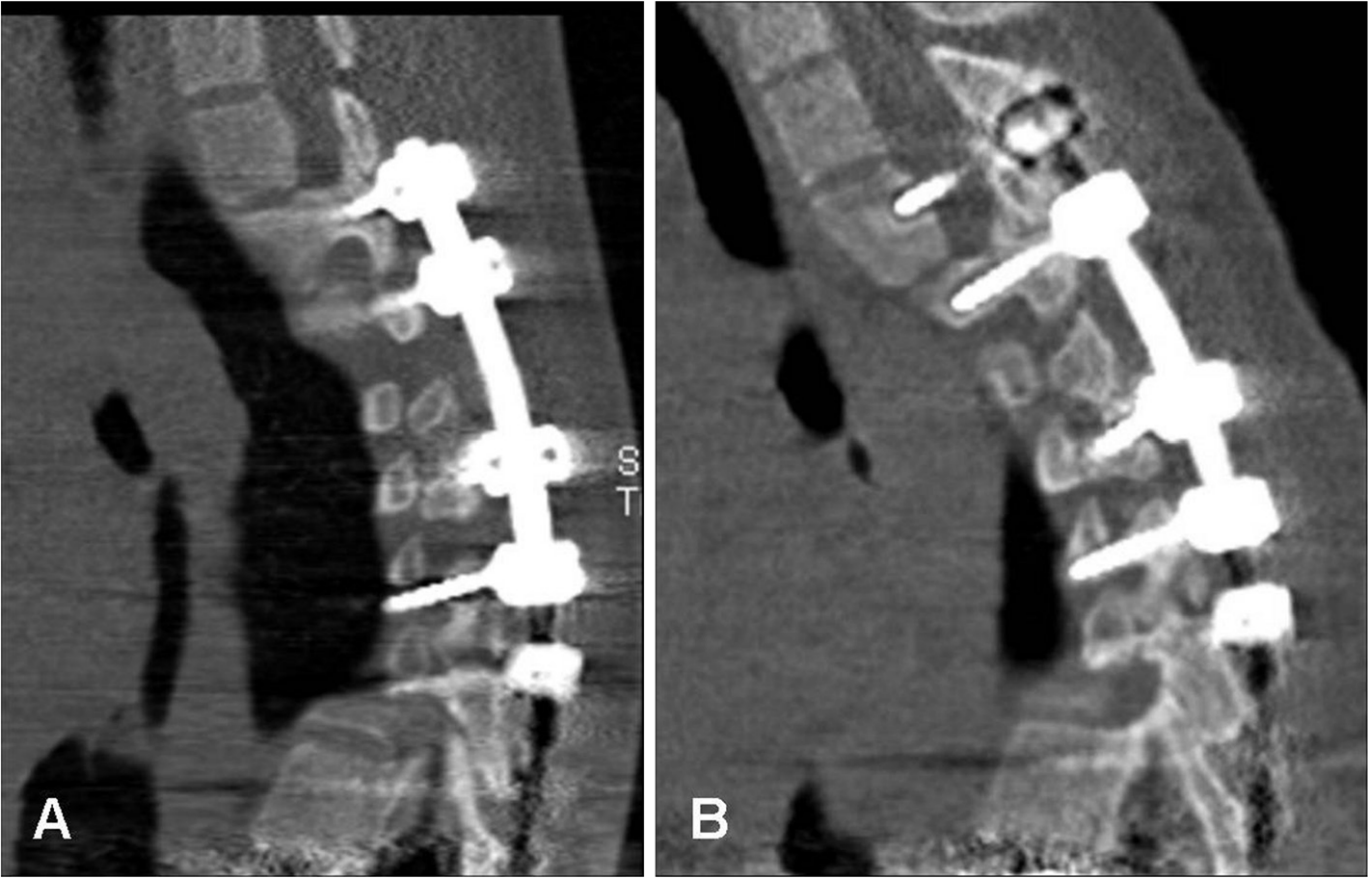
Evidence of screw loosening and proximal junctional kyphosis. CT of a 16 years old girl following segmental pedicle screw fixation T2-T12. Sagittal images (A) six weeks, and (B) two years following surgery of the same patient in Figure 2. Beside evidence of loosening, image B shows occurrence of proximal junctional kyphosis above the upper limit of the implant compared with image A.
